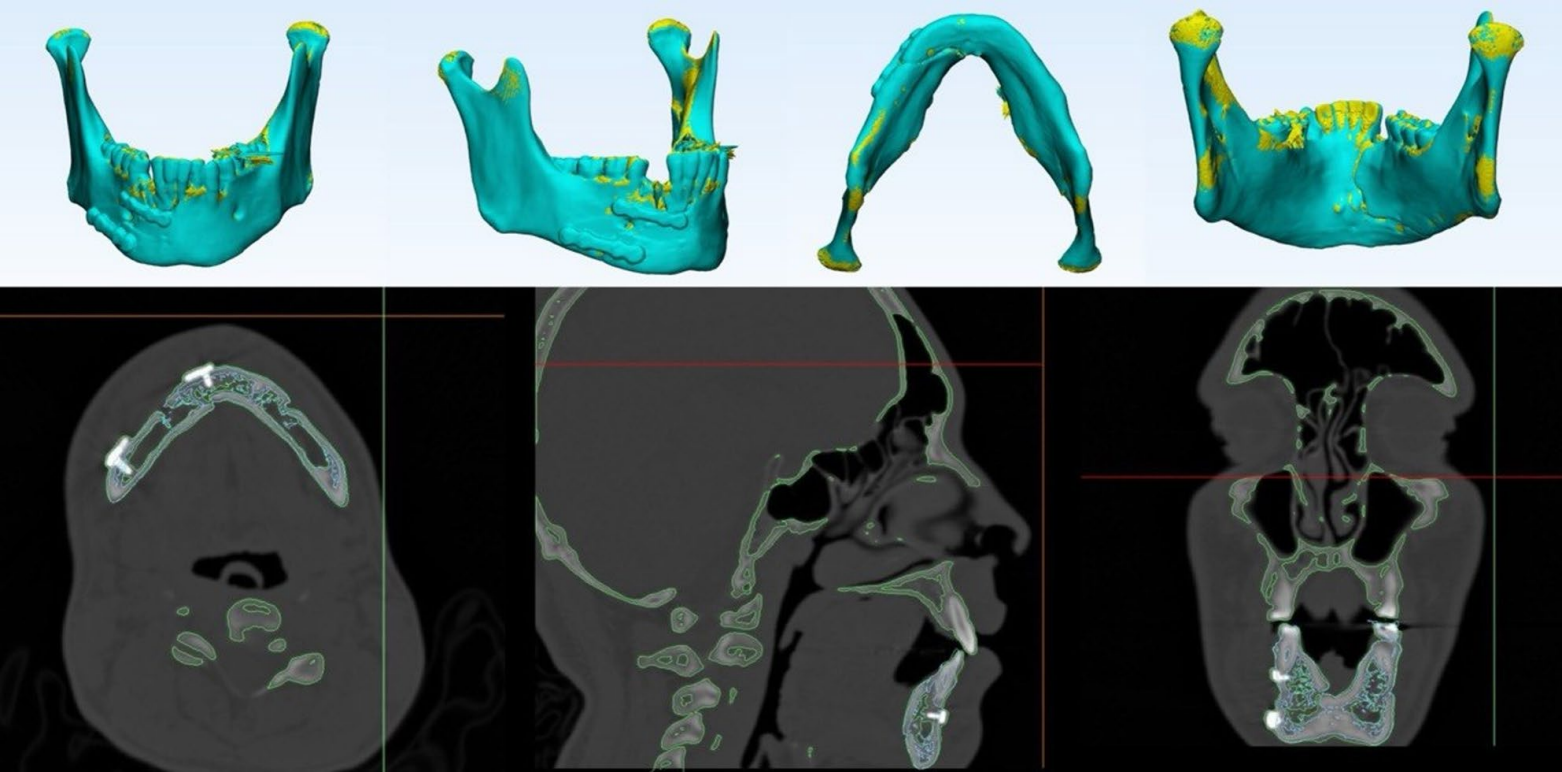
Snapshot of multiplanar Mimics (Materialise NV) screen showing the post operative radiographic assessment through super imposition of the actual post operative CT image over the virtual corrected CT image
